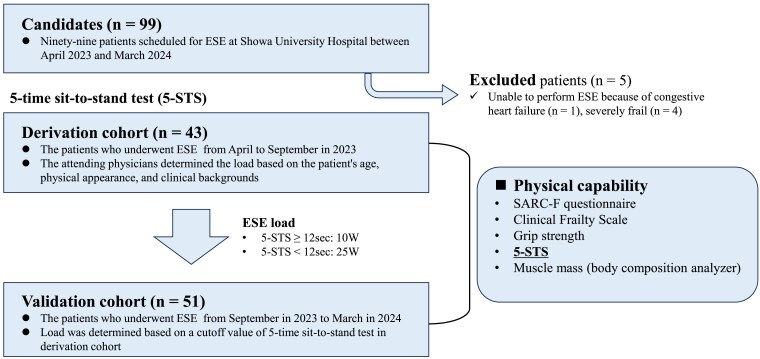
Patient enrolment. Of the 99 patients scheduled for exercise stress echocardiography at the Showa University Hospital from April 2023 to March 2024, 94 were eligible for analysis and were divided into two cohorts: derivation (*n* = 43, age/visual assessment-based load) and validation (*n* = 51, five times sit-to-stand test-based load) cohorts.
